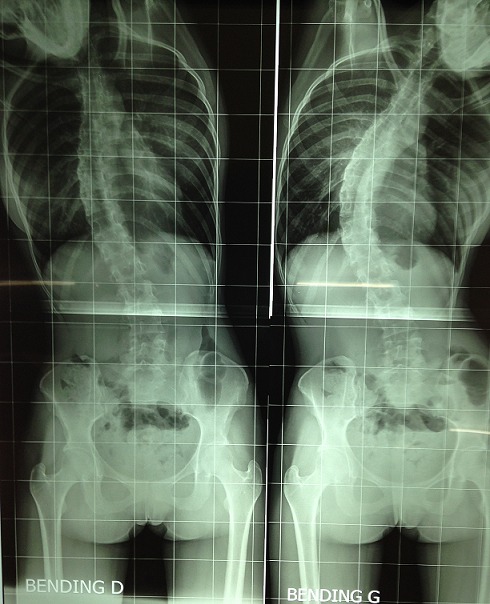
Clichés radiographiques préopératoires montrant la scoliose et le morphotype
